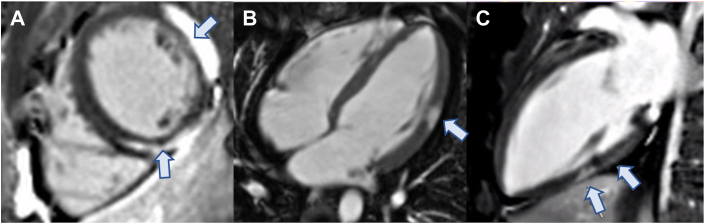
Initial Cardiac Magnetic Resonance Images on Presentation (A to C) Cardiac magnetic resonance images suggestive of myocarditis, with subepicardial late gadolinium enhancement in the midlateral wall and midwall late gadolinium enhancement in the midinferior wall (arrows).
